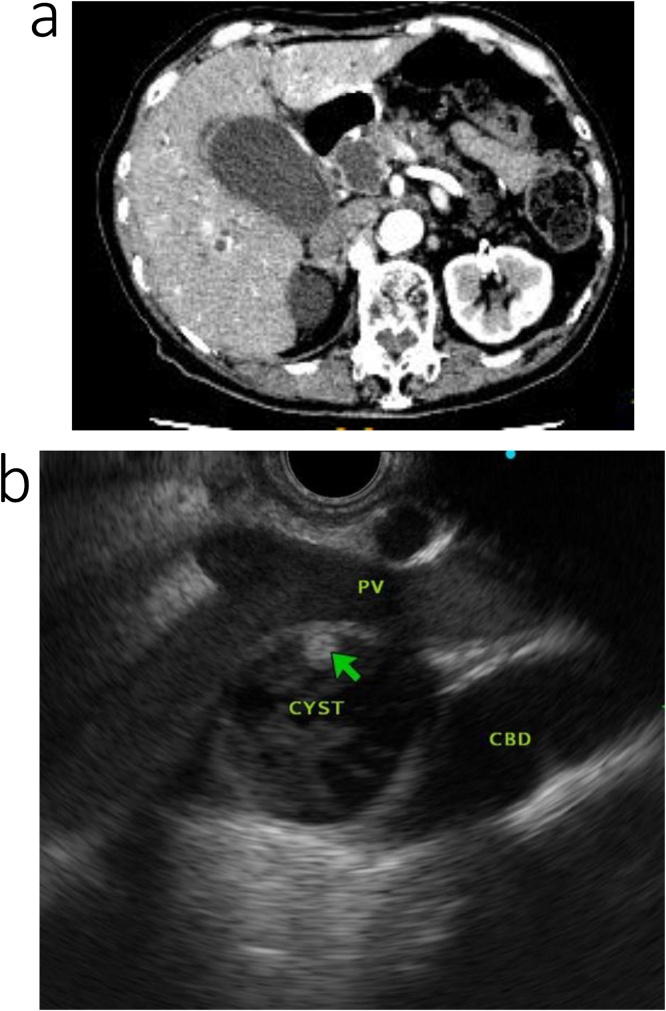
Contrast-enhanced computed tomography (CT) and endoscopic ultrasonography (EUS) for the patient in case 2. a. Contrast-enhanced CT images revealed dilation of the bile duct and of the common hepatic duct, with the contrast effect revealing a 26-mm cystic tumor in the head of the pancreas and mural nodules. b. A cystic tumor with mural nodules was found in the pancreatic head.
